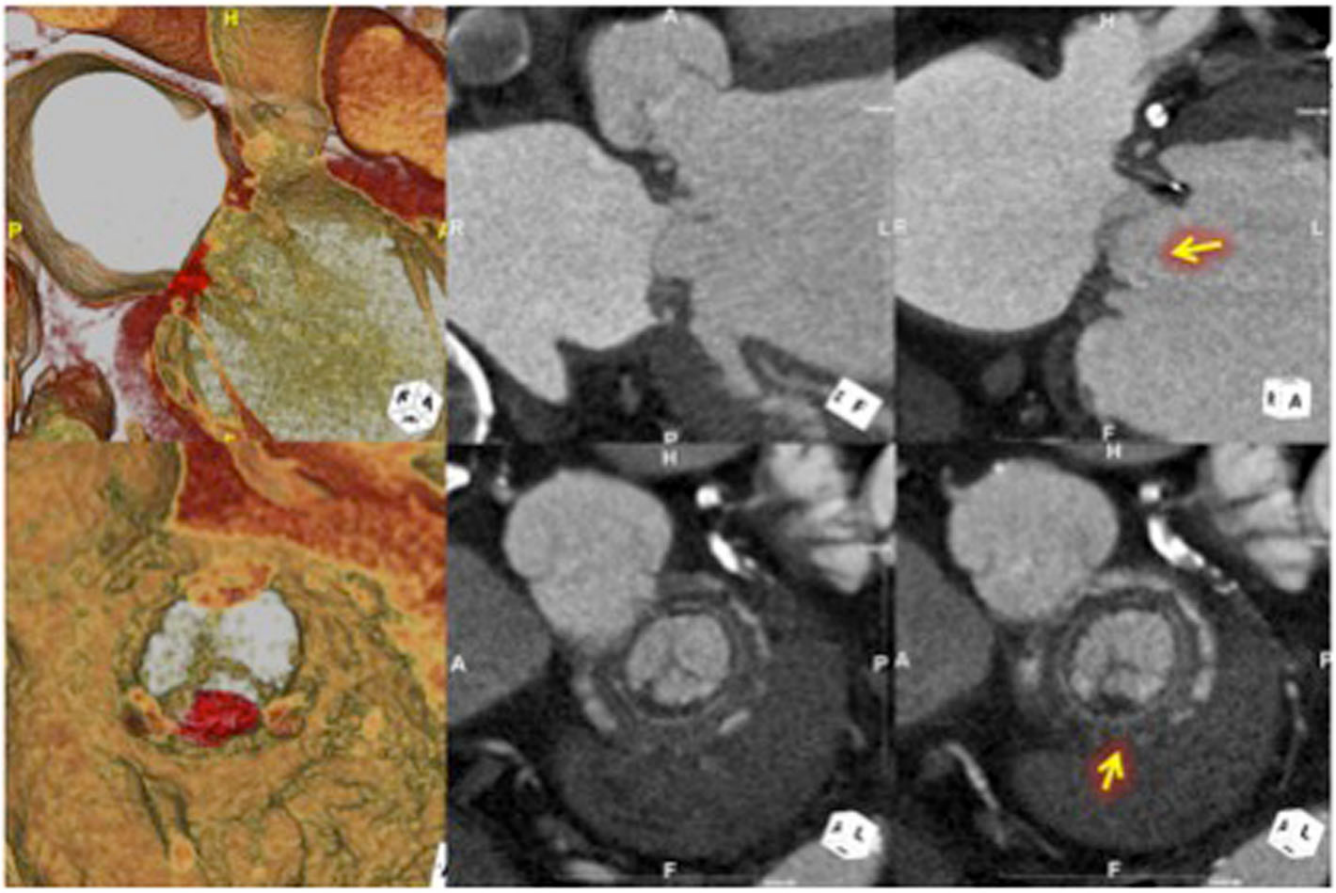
72-year-old male. 4 years out of mitral and aortic valve replacement. CT showed a HALT extending to almost the entire leaflet
